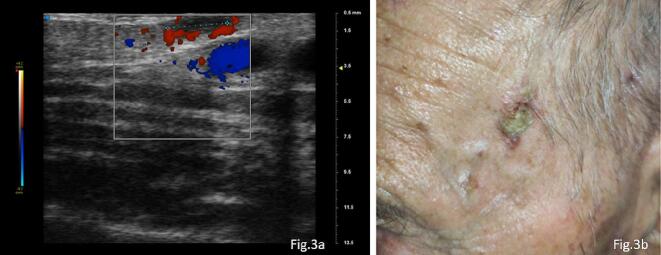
The UHFUS examination showed an oval shape with well-defined margins and a hypoechogenic structure with a thin signal on colour Doppler imaging (Fig. 3a). At the last follow-up, the lesion had grown significantly since the previous visit, it was excised and identified as SCC (Fig. 3b).
